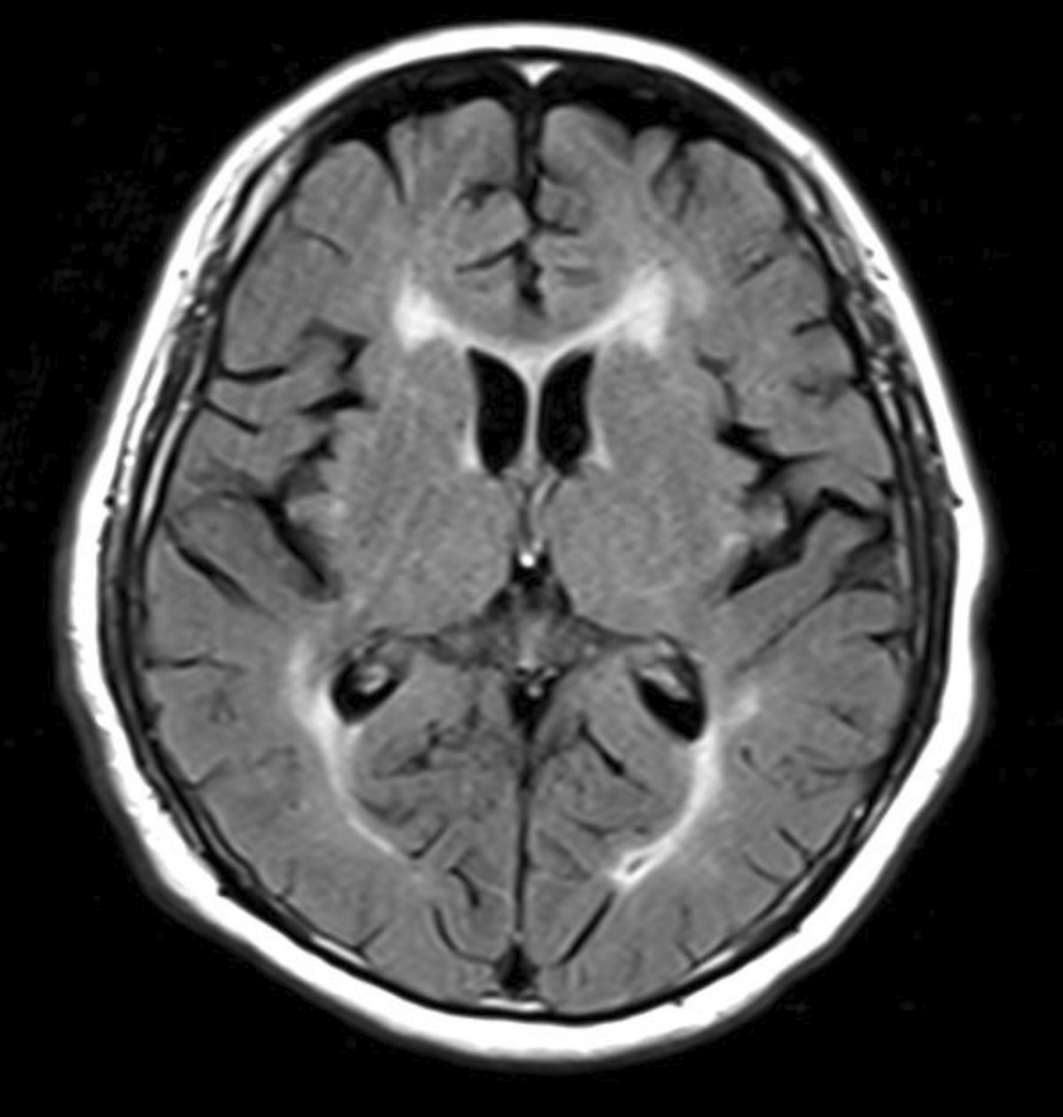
Head magnetic resonance imaging: There were no findings indicating new‐onset infarction.
